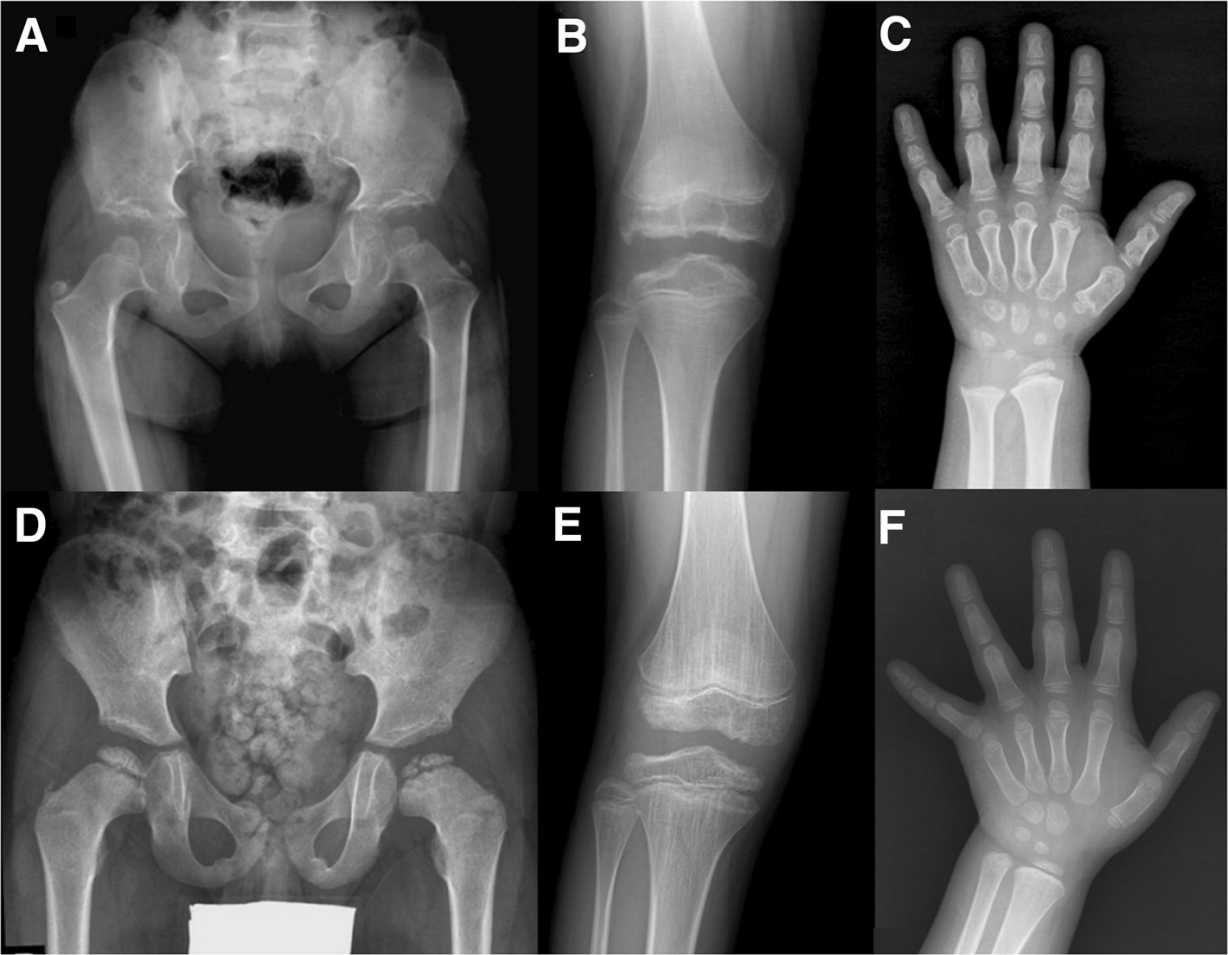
Radiographic features of COMP-MED (A, B, C) and of MATN3-MED (D, E, F). A: At 6 years of age, hip radiograph shows small and round femoral heads with irregular acetabular roof. B: At 12 years, the epiphysis at the knee joint is irregular and flattened. C: At 6 years, the hand radiograph shows brachymetacarpals, small and irregular carpal bones and distal radial epiphysis. D: At 6 years, the hip radiograph shows crescent shape femoral head with fragmentation and smooth acetabular roof. E: At 11 years of age, the vertical striations at the metaphysis are conspicuous along with irregular and flattened epiphysis. F: At age 6 years, the hand radiograph shows no brachymetacarpals or carpal bone irregularity. (This figure is partly a citation for images taken from “Revisit of Multiple Epiphyseal Dysplasia: Ethnic Difference in Genotypes and Comparison of Radiographic Features Linked to the COMP and MATN3 Genes” of the American Journal of Medical Genetics Part A. It is in the Nov 2011, Volume 155A, Issue 11:2669–2680 with permission).
